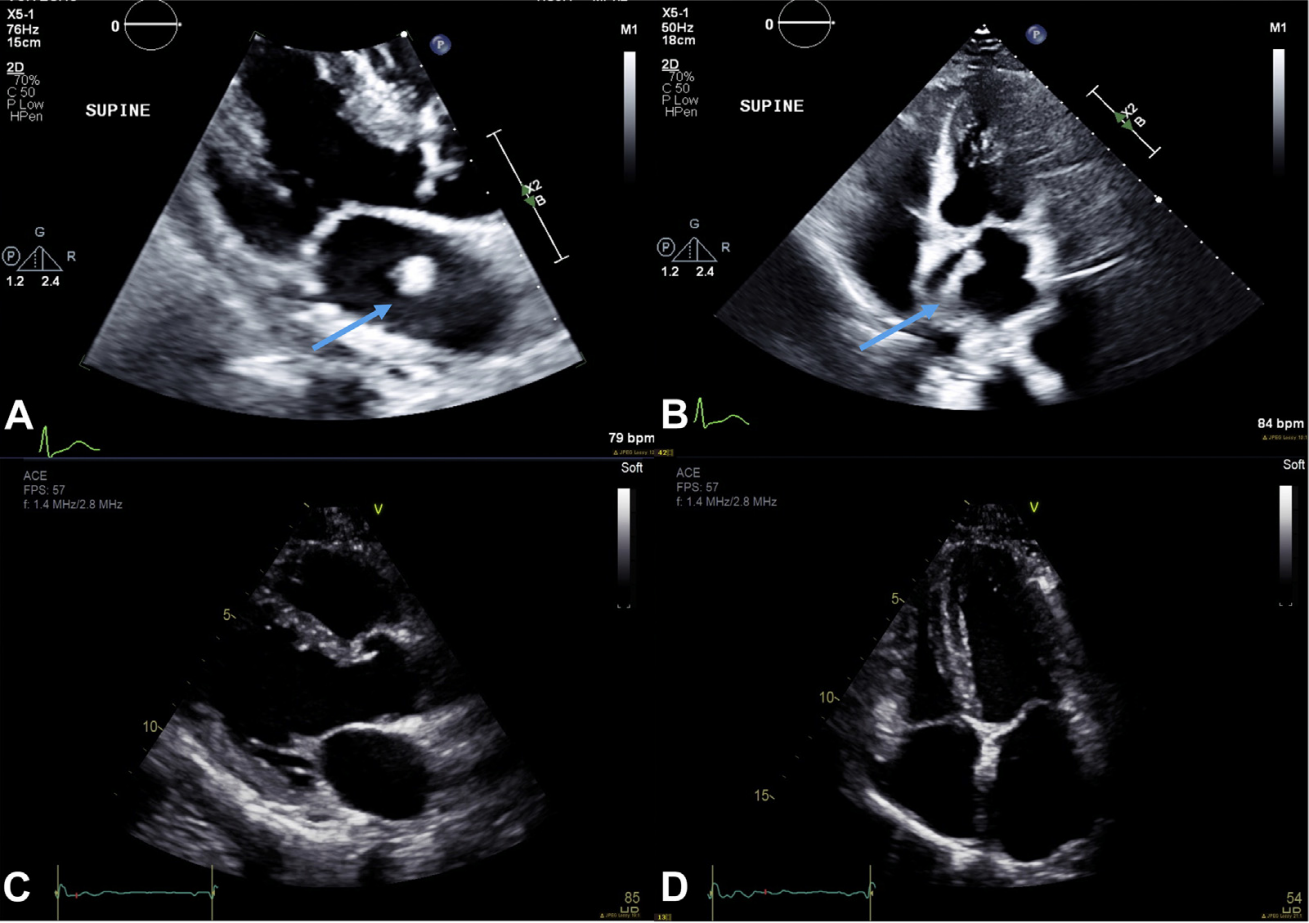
Transthoracic echocardiogram showing the parasternal long-axis *(left)* and 4-chamber views *(right)*. (A-B) Initial TTE with pedunculated stalk-like mass *(blue arrows)* with maximal orthogonal dimensions of 15 × 35 mm in the apical 4-chamber view and 15 × 12 mm in the 2-chamber views. (C-D) Transthoracic echocardiogram 12.5 weeks postdiagnosis and 10.5 weeks of therapy with no LA mass identified.
